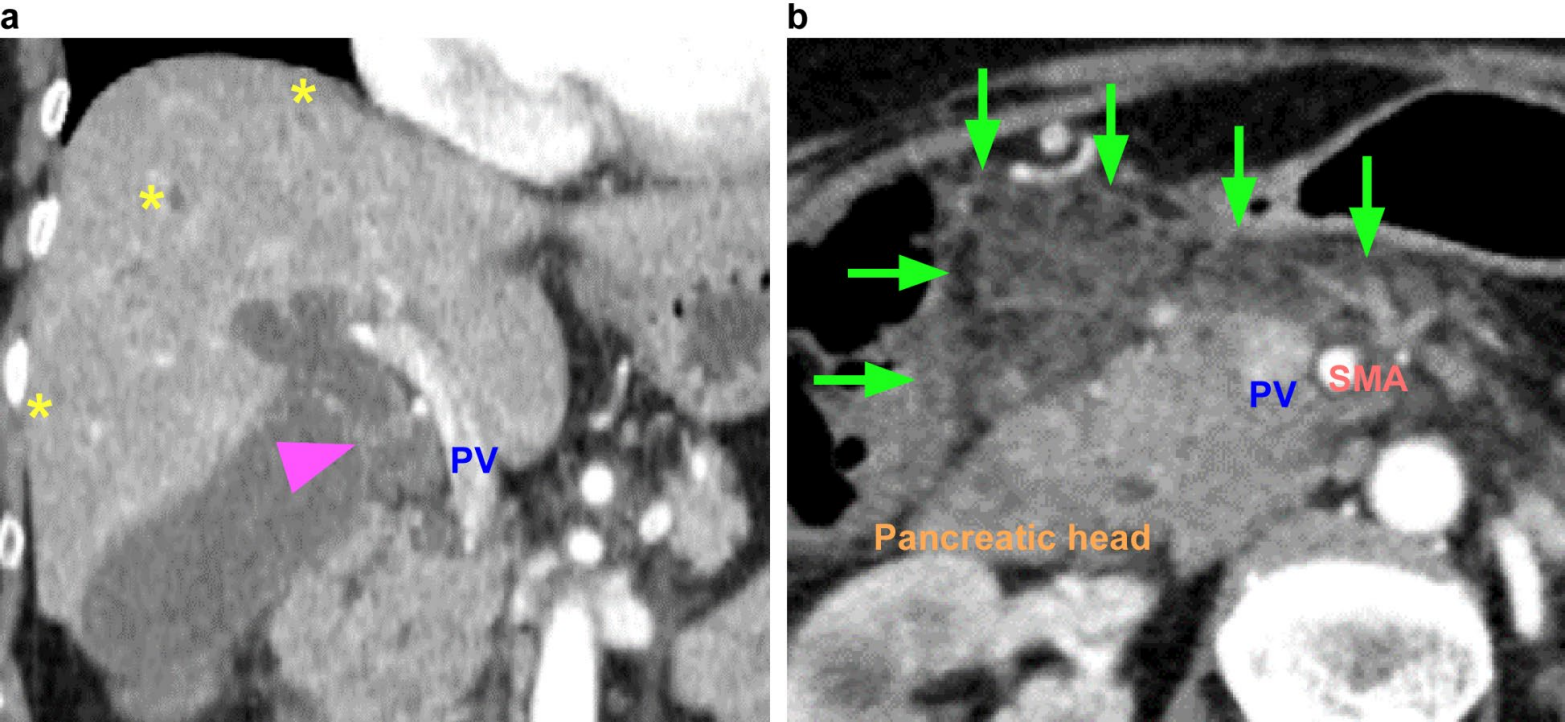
CT images of the tumor and pancreatitis after endoscopic retrograde cholangiopancreatography. a Enhanced tumor (arrowhead) is seen in a distal bile duct. The intrahepatic bile duct is mildly dilated (+). b Extensive inflammation (arrows) is present from the pancreatic head to the mesentery of the transverse colon
